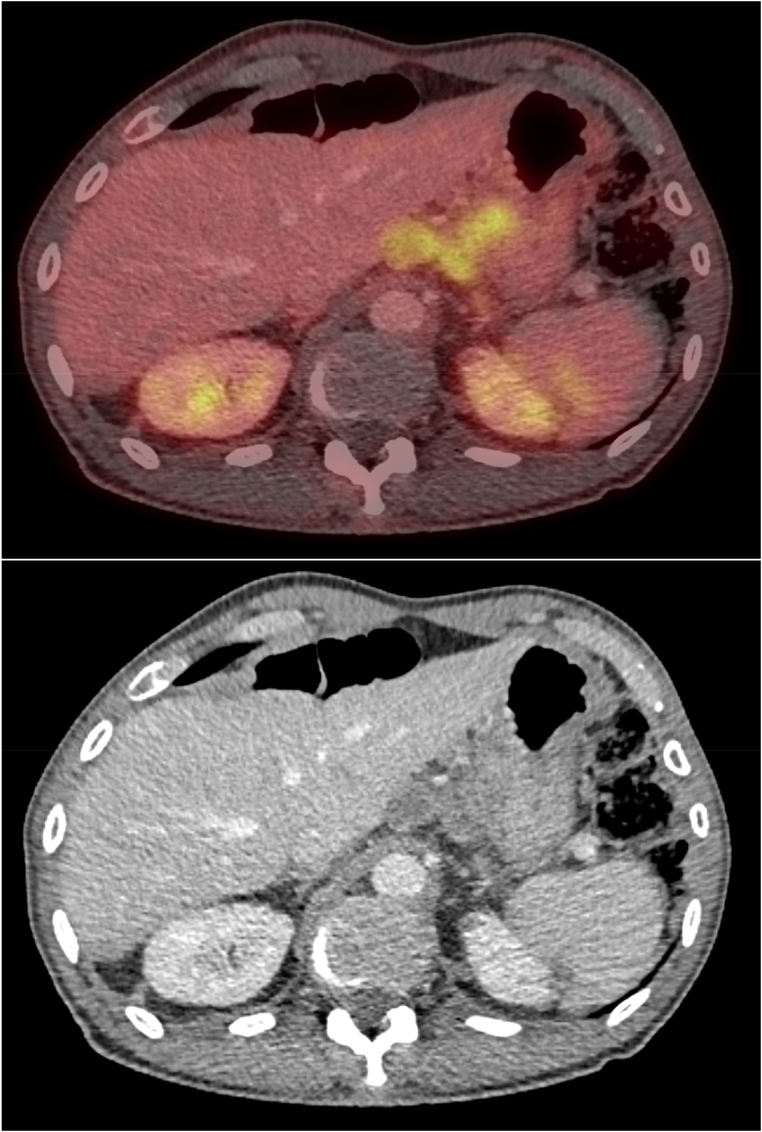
Siewert Type 2 left gastric nodes on PET/CeCT (CeCT = contrast-enhanced CT; CT = computed tomography; PET = positron emission tomography)
